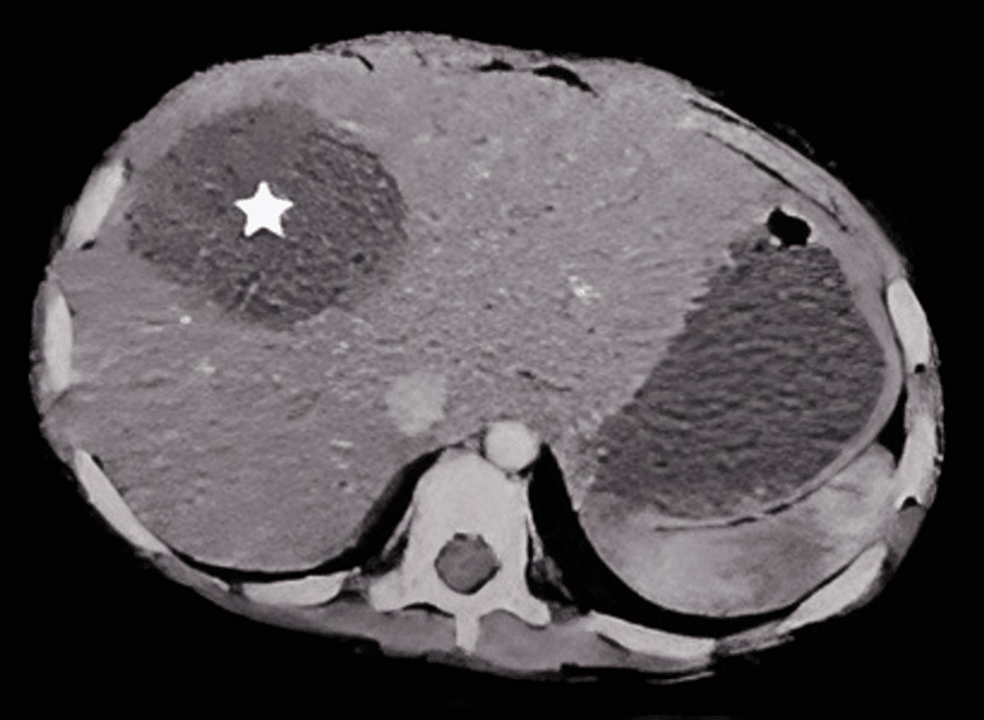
CT showing a 4x2 cm cystic lesion (asterisk) in the liver.
